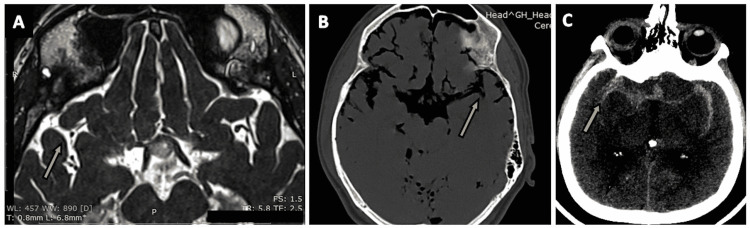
The Sylvian cistern (continued). (A) The Sylvian cistern (arrow) as seen on an axial section of the routine three-dimensional (3D) constructive interference in steady state (CISS) MRI sequence. (B) The Sylvian cistern (arrow) as seen on an axial section of CT brain (negative contrast) in a case of pneumocephalus. (C) The Sylvian cistern (arrow) as seen on an axial section of CT brain (positive contrast) in a case of subarachnoid hemorrhage (SAH).
